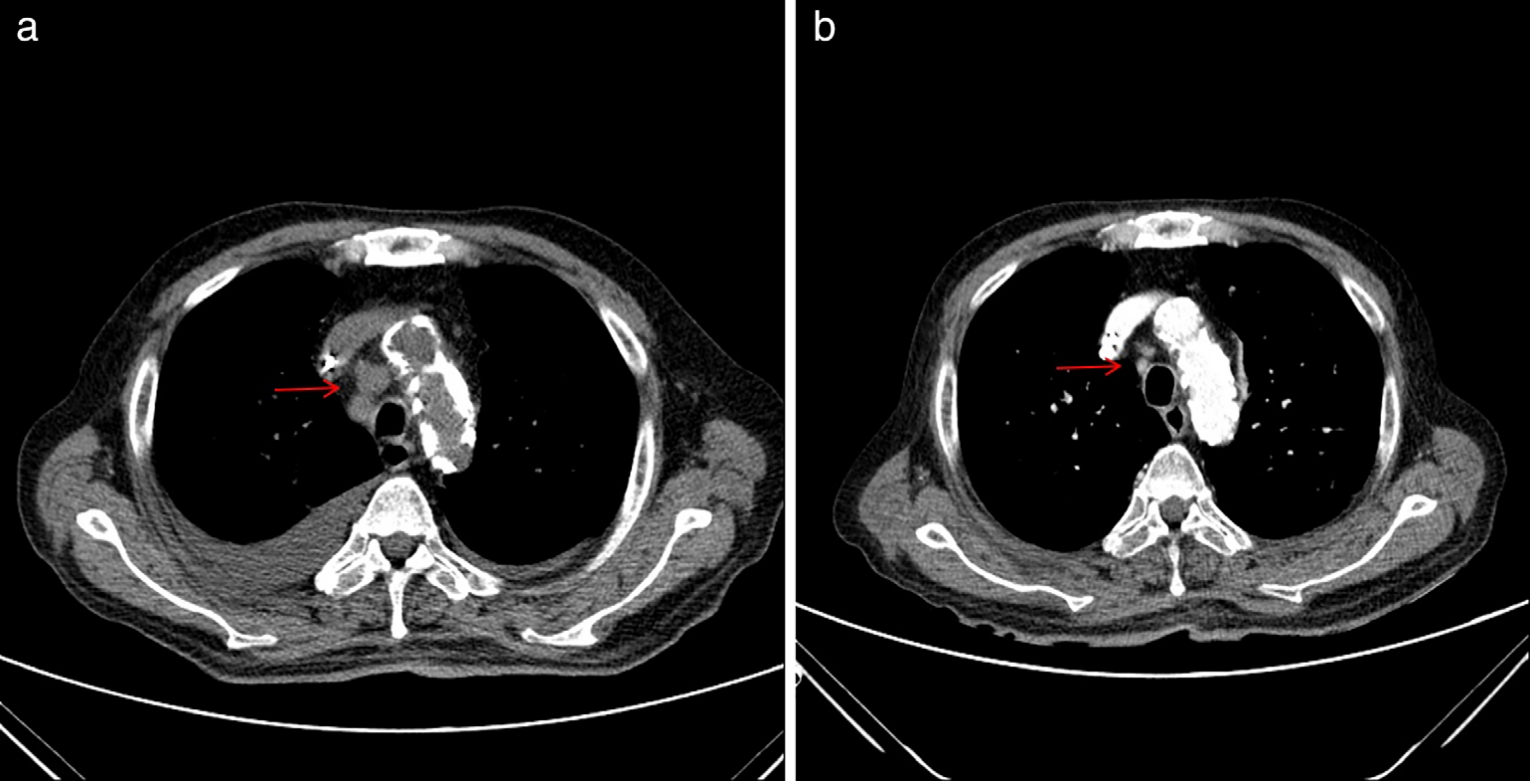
Chest computed tomographic (CT) scans. (a) The chest CT scan showed enlargement of multiple mediastinal lymph nodes. (b) After one month of crizotinib treatment, mediastinal lymph nodes were found to be markedly regressed.
